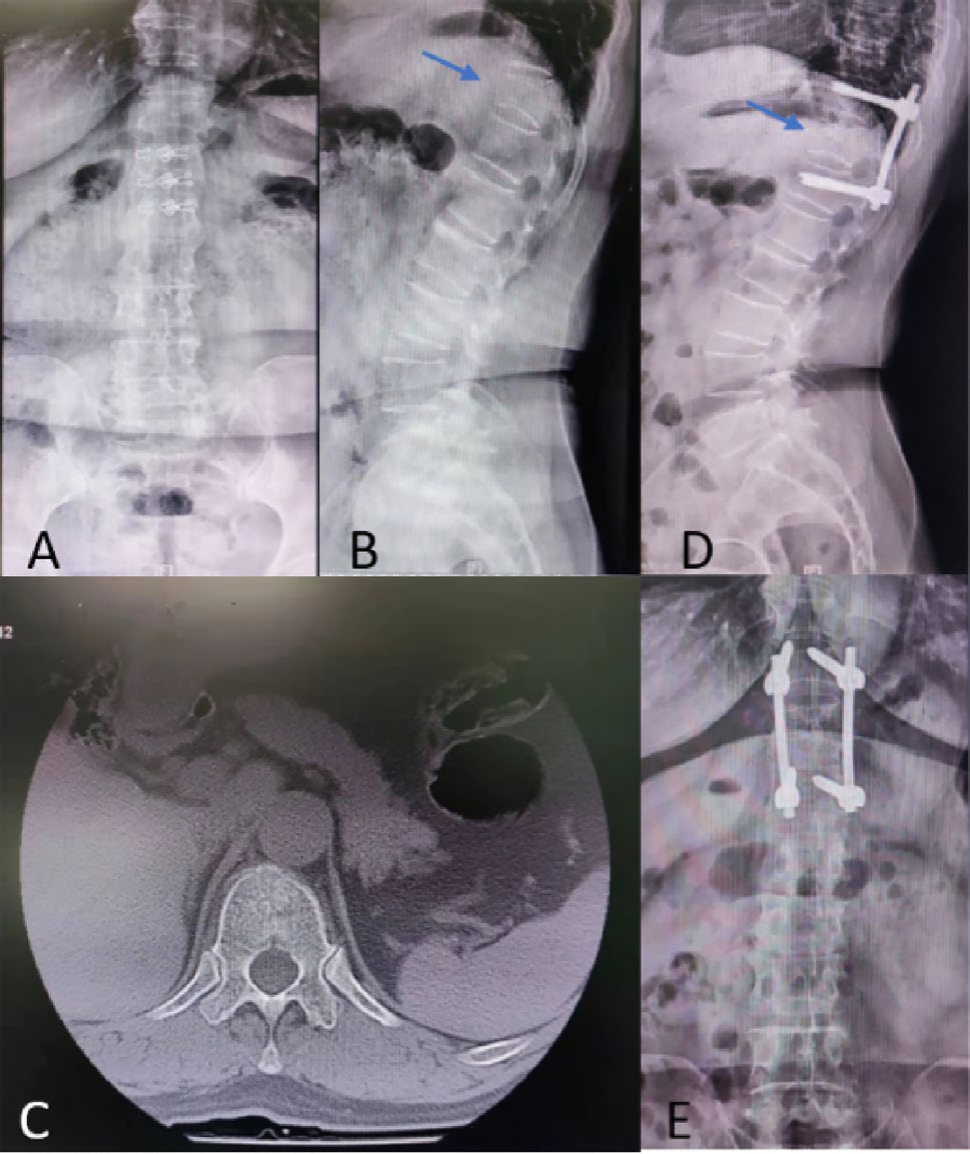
A case of AO A1.3 fracture patient. Preoperative X ray (A, B) and CT scan (C) and postoperative X ray (D, E).
